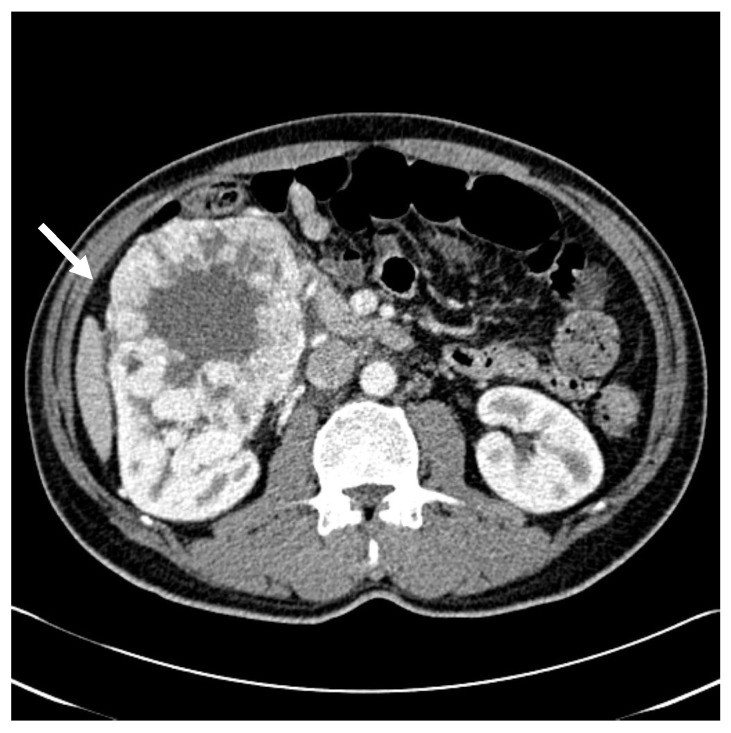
Initial abdominal CT showed a lobulated, heterogeneously and avidly enhancing mass measuring up to 14.3 cm in maximal diameter, located in the anterior portion of the right kidney’s lower pole (white arrow). The lesion exhibited internal necrosis and contained multiple calcific foci.
